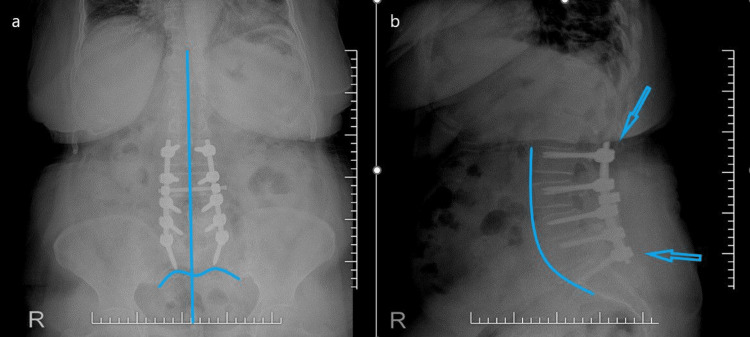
Posterior lumbosacral instrumentation (a) anteroposterior and (b) lateral digital radiographs. The blue vertical line shows the central sacral vertical line, the blue curved line shows the lumbar lordosis, and the arrows show the pedicle screws and rods.
